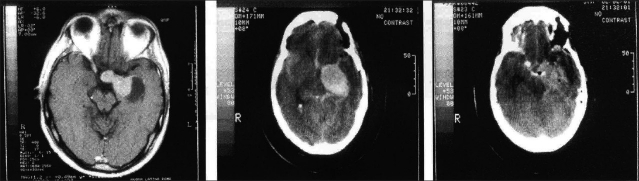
a) Axial T1 scan shows a pituitary tumor with significant left lateral extra-sellar extension. b) Immediate postoperative CT scan shows a huge hematoma. c) CT scan following re-operation demonstrates partial removal of the mass
